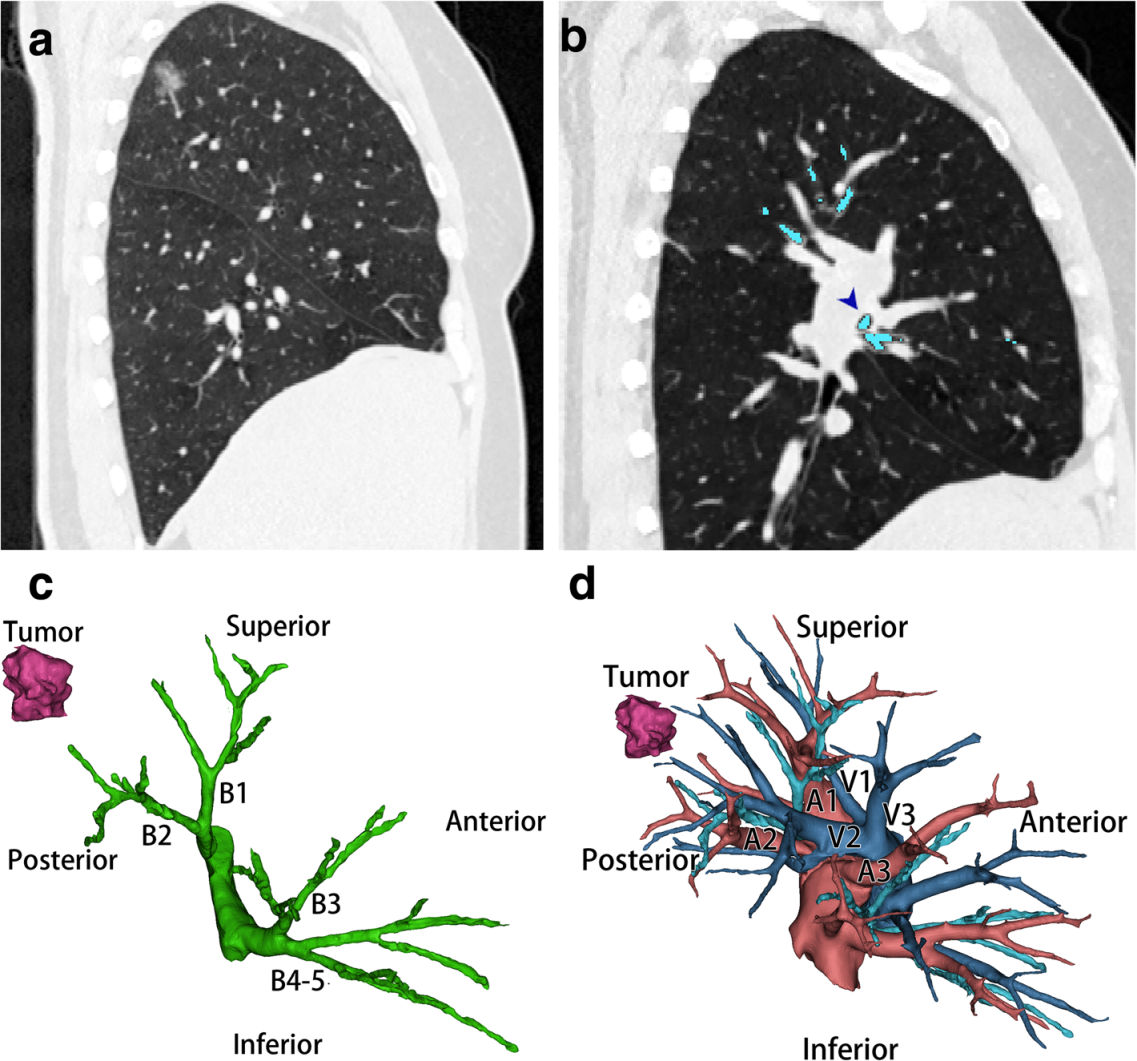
Preoperative computed tomographic and 3-dimentional computed tomographic bronchographic and angiographic images. a sagittal image showing a nodule resides in the apical-posterior segment. b sagittal image showing the anterior segmental bronchus derives from the middle lobe bronchus (arrow). c The distribution of right upper and middle lobe bronchi, noted that the anterior segmental bronchus derived from middle lobe bronchus. d The distribution of right upper and middle lobe vessels, noted that the anterior segmental pulmonary artery branched from the pulmonary trunk distally to the branching sites of apical and posterior arteries. The distribution of pulmonary vein is relatively normal
